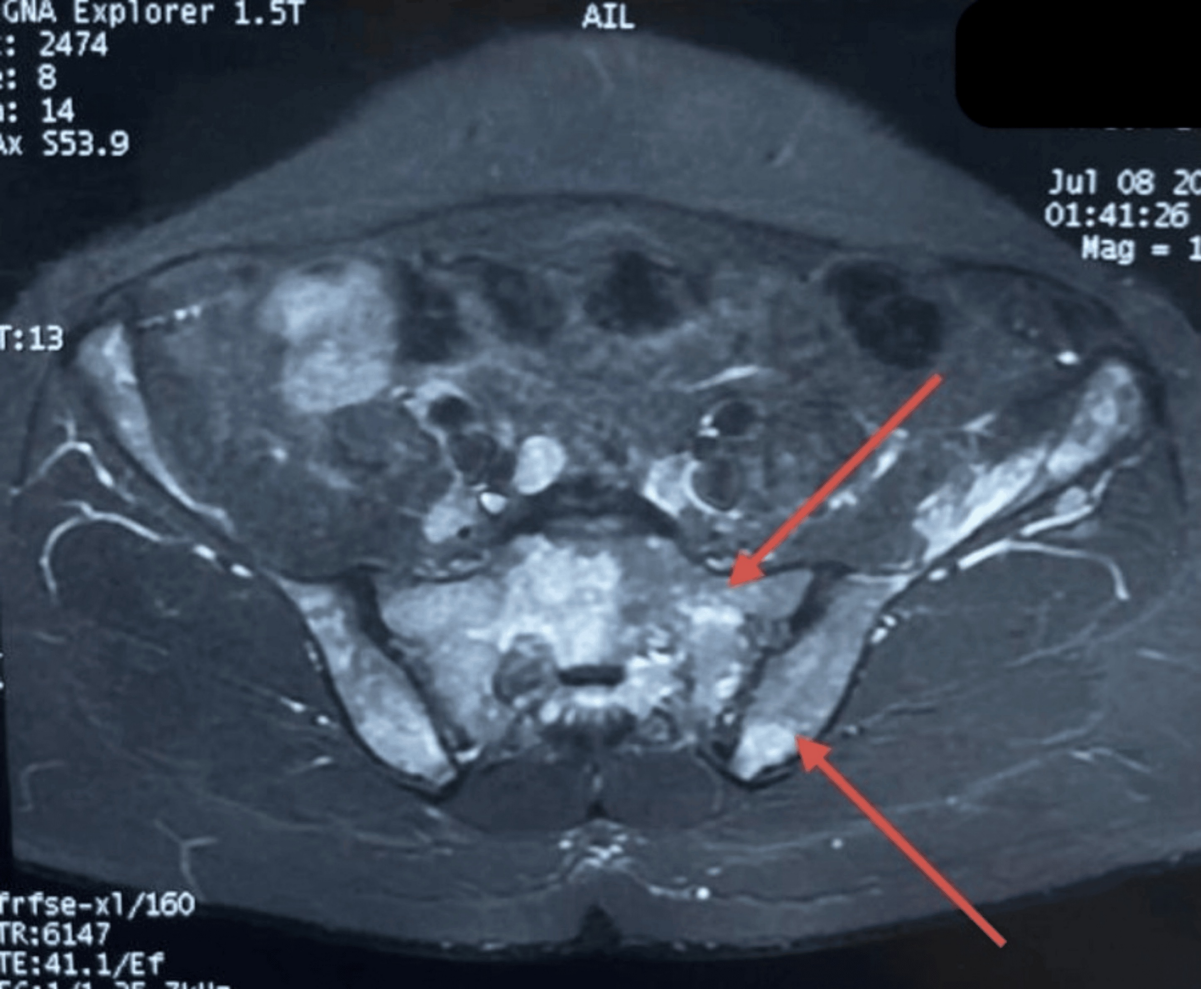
Pelvic MRI Pelvic MRI (coronal STIR sequence) showing bilateral sacroiliac arthritis with diffuse bone marrow hyperintensity involving the pelvic girdle and femoral heads. Cortical integrity is preserved. STIR - short tau inversion recovery
